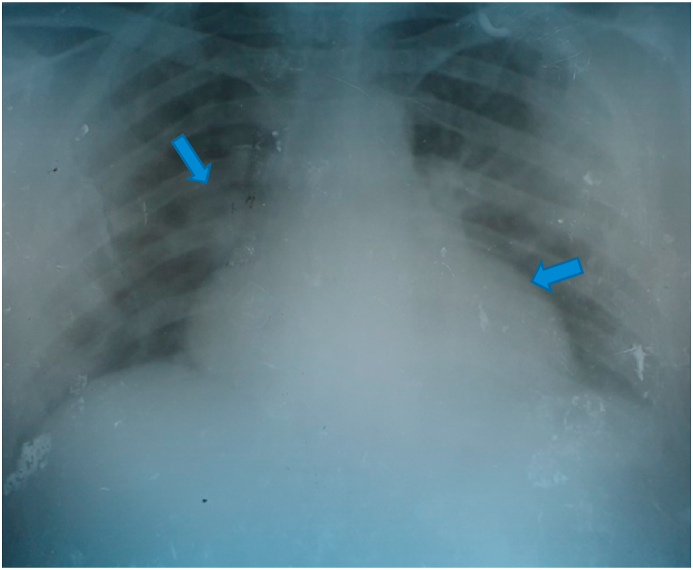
Chest xray revealing cardiomegaly and bilateral lung opacities.
